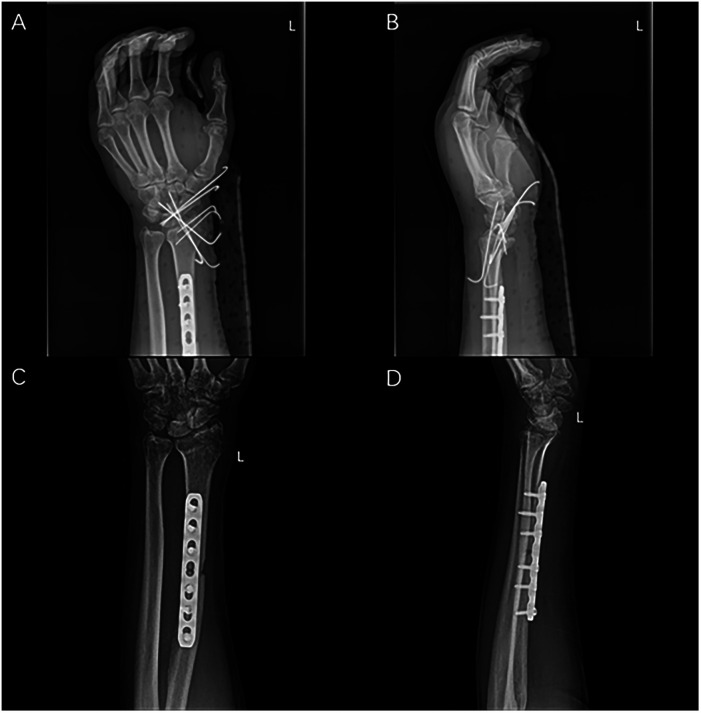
Post-operative radiographs. Anteroposterior radiographs at 3rd day (A,B) and at 6th week (C,D).
